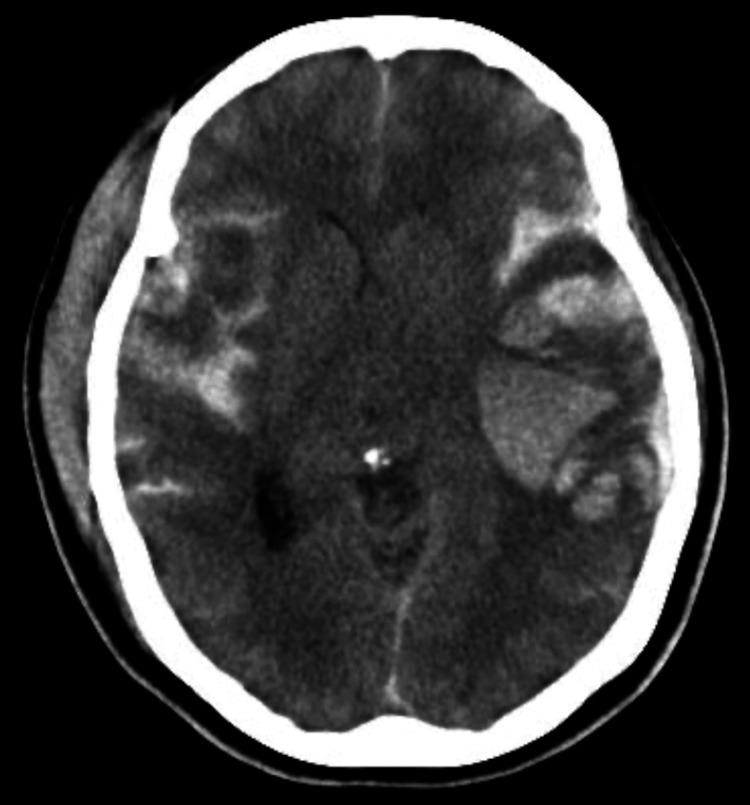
Head computed tomography (CT) scan two hours after arrival Two hours later, the CT scan showed an enlarged contusional hematoma in the left cerebrum and a midline shift.
